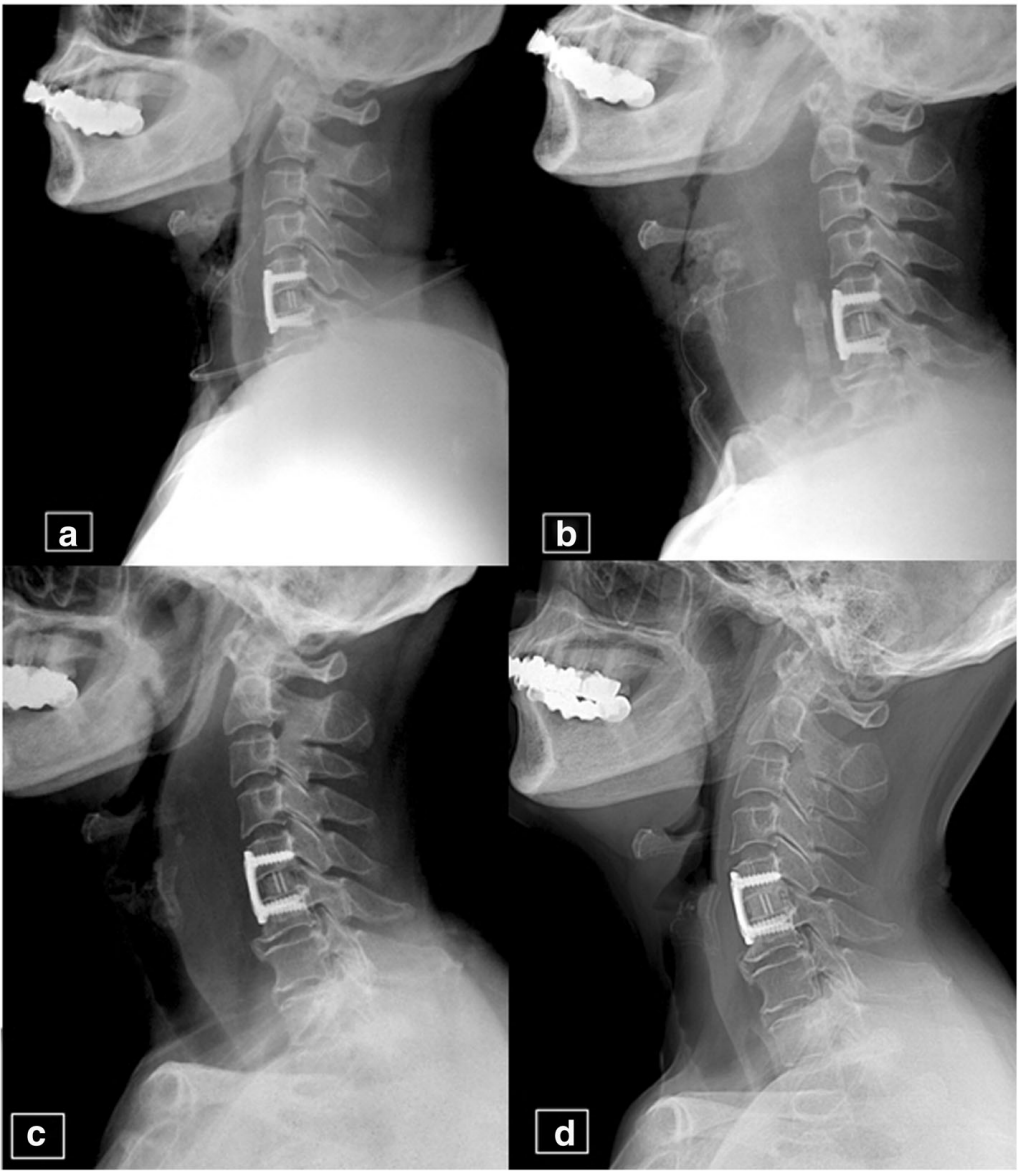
A 67-year-old female patient. a Lateral plain radiograph of the cervical spine on the day of operation showing anterior cervical discectomy and fusion at C5-6. b Lateral radiograph 12 h postoperatively during acute respiratory failure showing severe prevertebral swelling. c Lateral radiograph at 10 days postoperatively showing decreased prevertebral swelling. The patient was discharged without complications. d Lateral radiograph at the 12-month follow-up showing no prevertebral swelling
